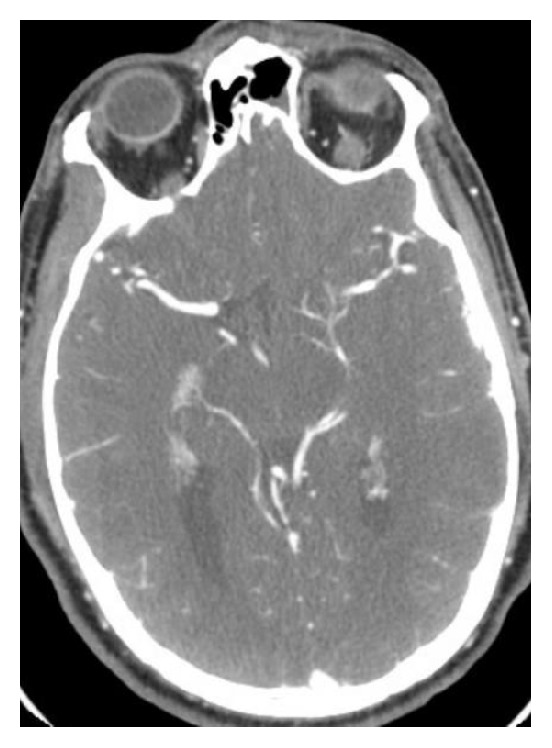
CT angiogram sequence of the left middle cerebral artery at the bifurcation with narrowing.
